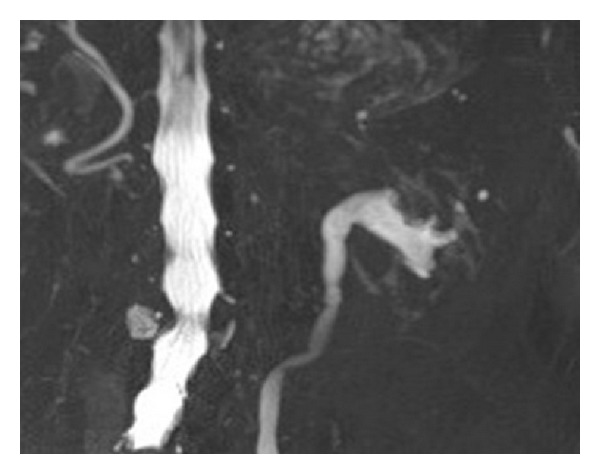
T2-weighted MR urography demonstrates a mass at the upper pole of the left kidney directly invading the renal pelvis.
